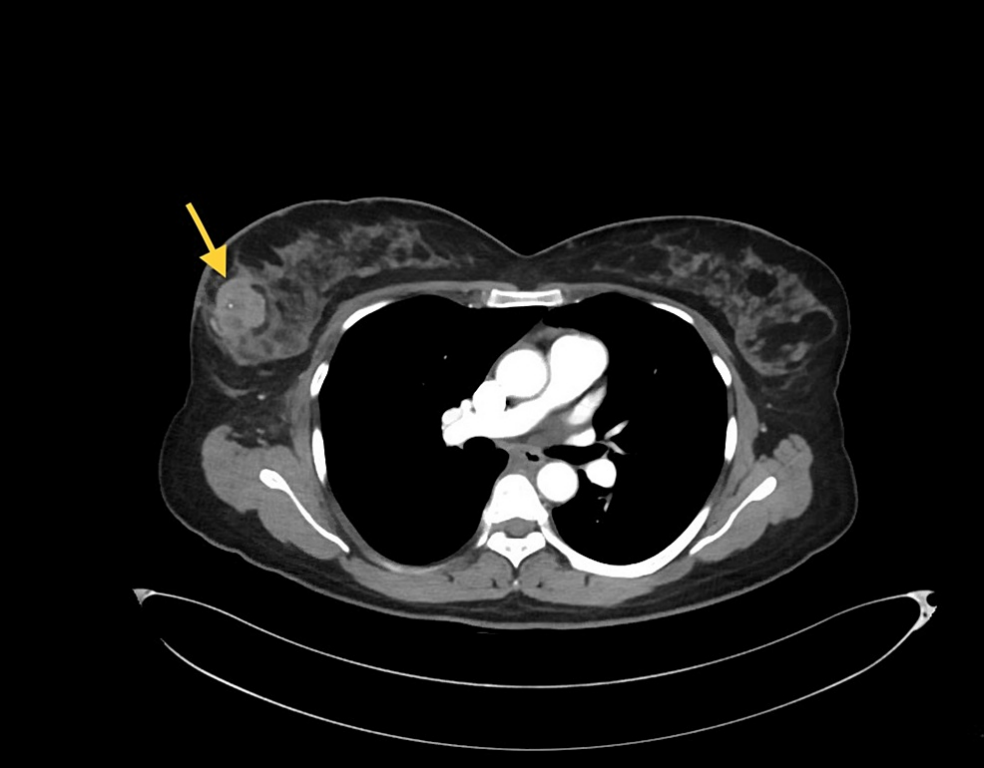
CT chest with intravenous contrast showing the lump in the right breast.
